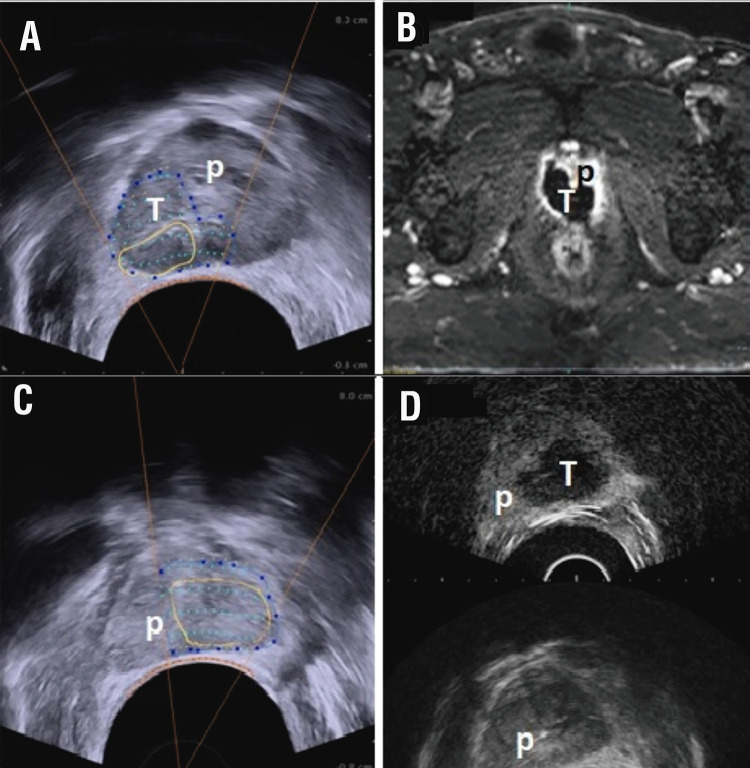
Contrast-enhanced ultrasound image after HIFU. A) Image fusion and treatment planning; B) Treated area control; p=prostate; T=treated area. C-D) (different patient from figures A-B). mpMRI image 1 month after HIFU (hemiablation) + TURP. Patient 77y, PSA: 7.5ng/mL; PCa Gleason 3+4; mpMRI: 10mm lesion 10p PIRADS 5/5, prostate volume 30mL; control PSA: 1.71ng/mL; p=prostate; T=treated area.
